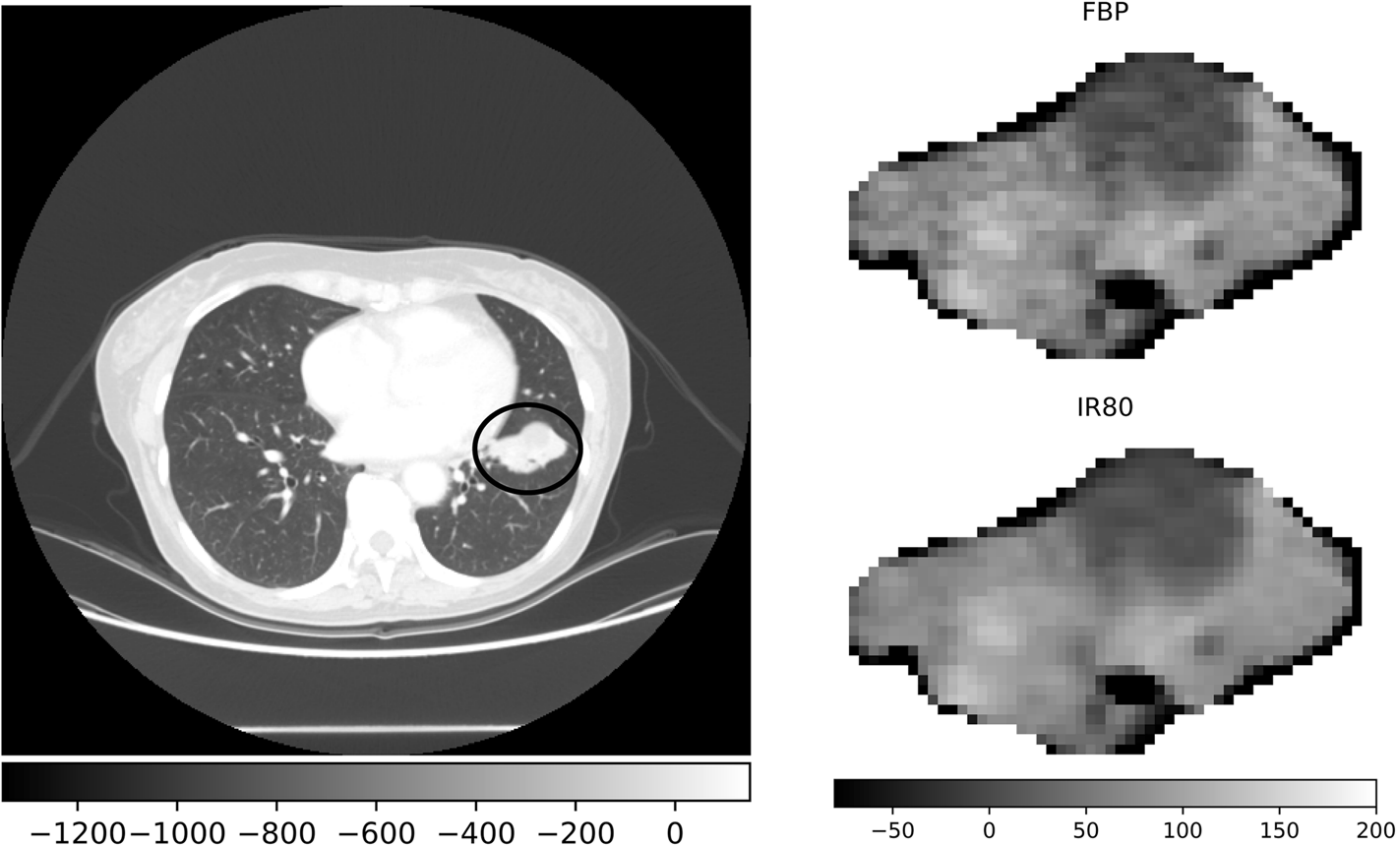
Visual comparison of computed tomography images of the same patient reconstructed with two blending levels. The image on the left shows the thorax of the patient with the encircled lesion, displayed using the lung window. The same lesion is isolated in the right figures, displaying the filtered backprojection (FBP) reconstruction and the iterative algorithm with ASIR 80% (IR80) with a mediastinal window
